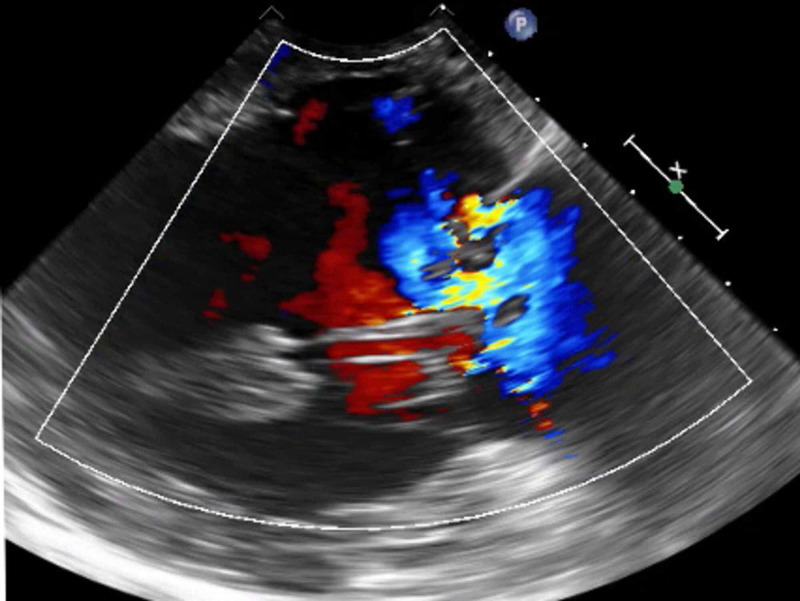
TEE confirmed flail anterior mitral leaflet and chordae due to posteromedial papillary muscle rupture, as well as severe posteriorly directed TEE: Transesophageal Echocardiogram
